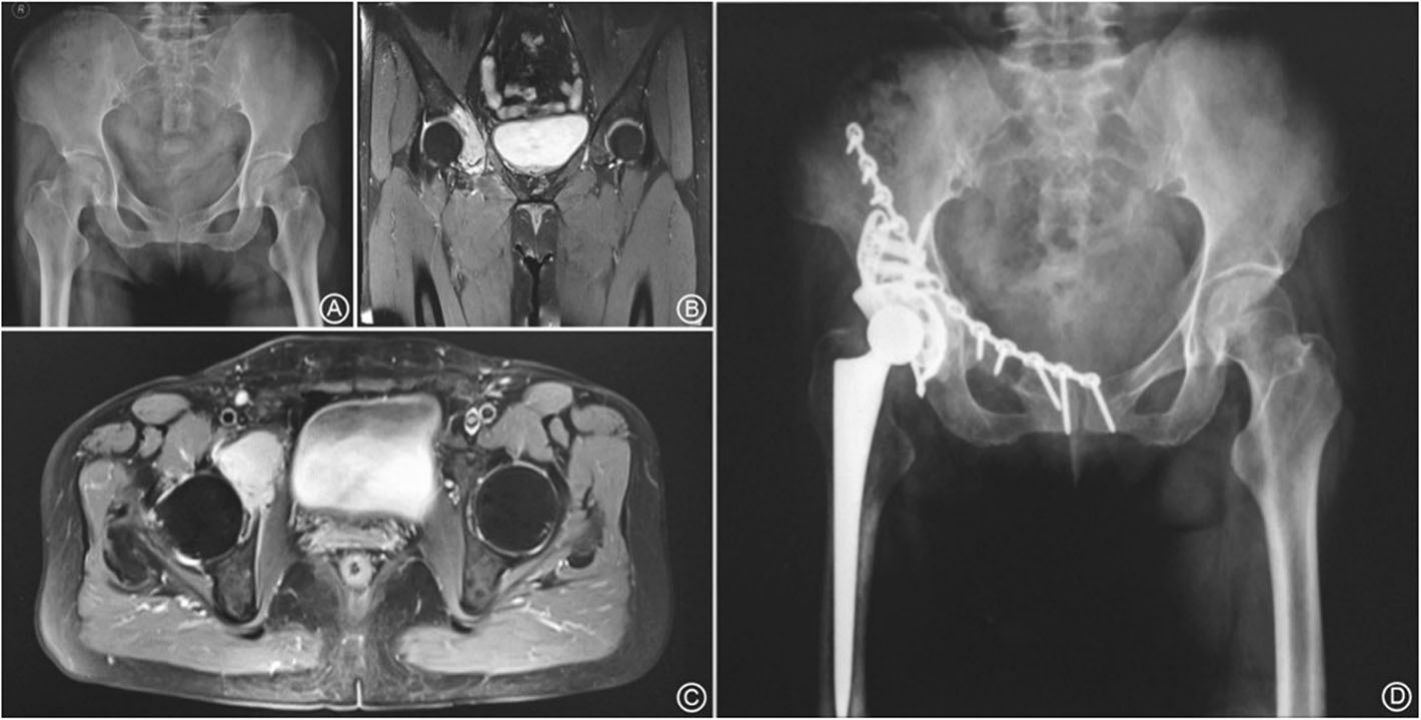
A 57y female patient diagnosed with Type C tumor [malignant giant cell tumor of bone (MBGCT)]. a. Preoperative pelvic X-ray shows a right ilium tumor with the involvement of the superior edge of the acetabulum; b. Preoperative coronal MRI shows enhanced signal on the superior edge of acetabulum; c. Preoperative cross-sectional MRI shows enhanced signal on the superior edge of acetabulum; d. 1y postoperative pelvic X-ray shows the reconstruction and prosthetic position
